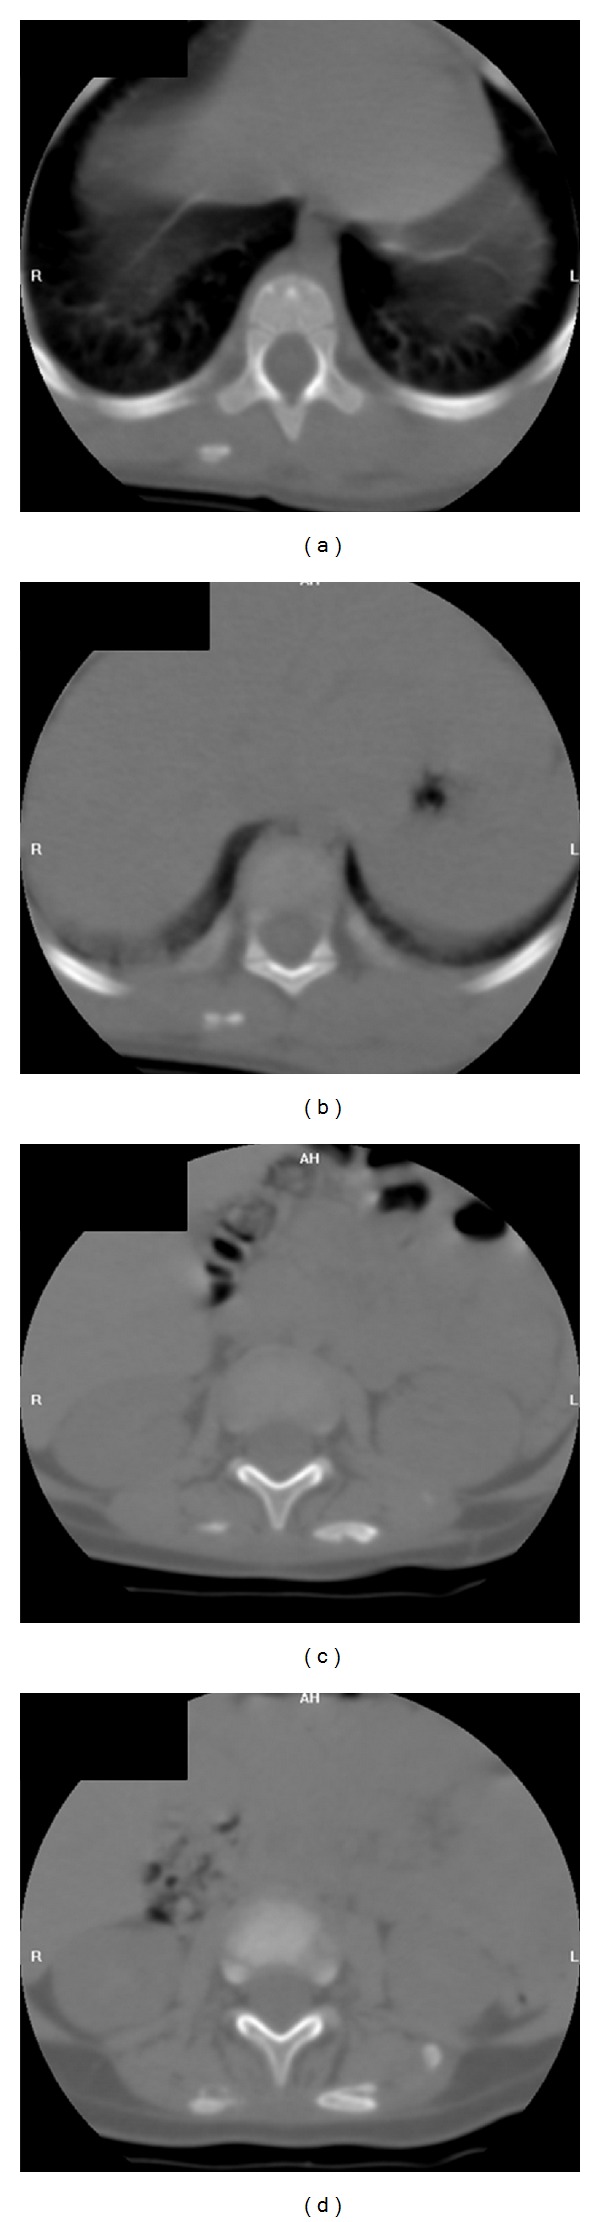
Noncontrast axial CT scan of lumbosacral spine showing multiple plaque-like calcifications in paraspinal muscles, more on the left side, with diffuse muscle thickening.
